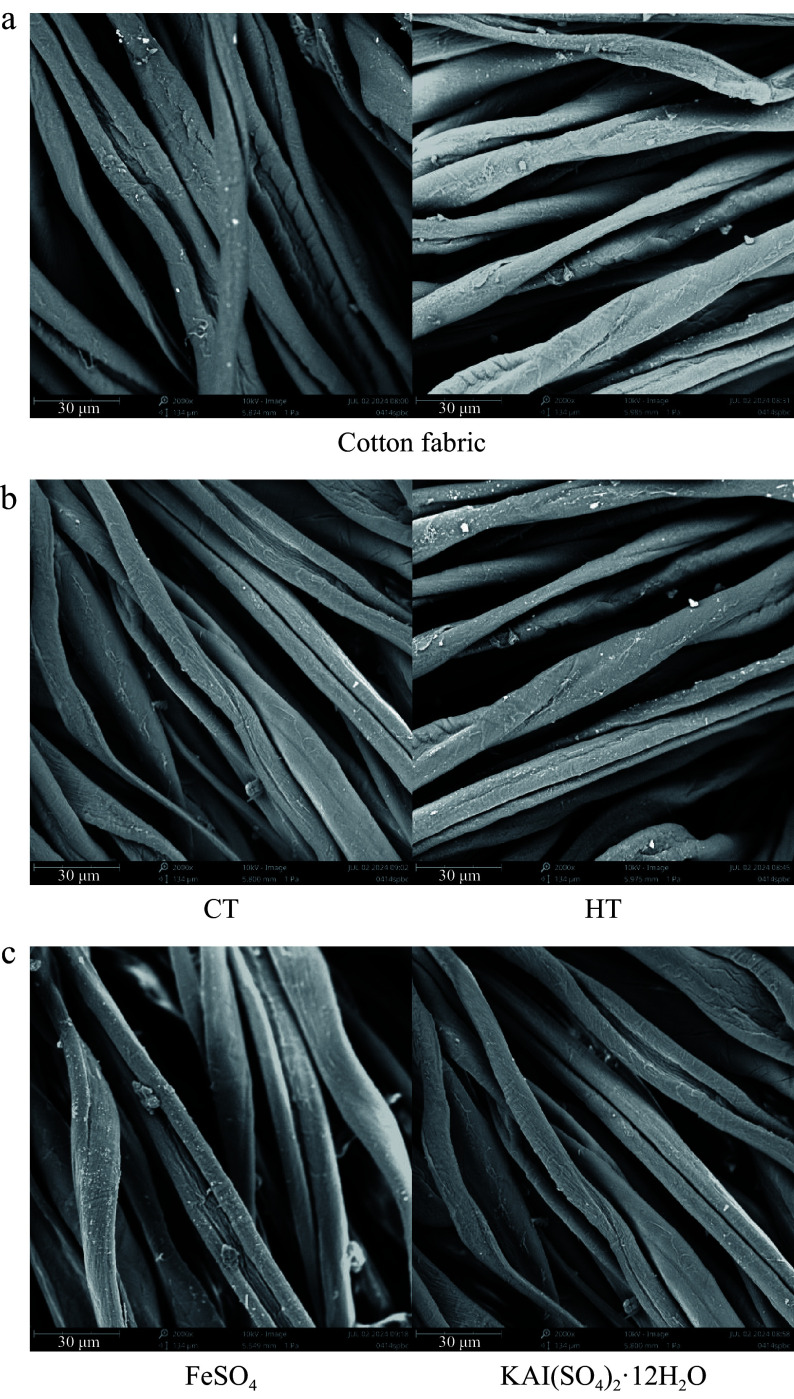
Surface morphology of cotton fabrics. (a) Surface morphology of untreated cotton fabric. (b) Surface morphology of cotton fabric directly dyed with different plant dye components from *Camphora officinarum* seeds. CT refers to the dye solution extracted using 60% ethanol as the solvent, with condensed tannins as the main component, while HT refers to the dye solution extracted using water as the solvent, with hydrolysable tannins as the main component. (c) Surface morphology of cotton fabric after post-mordanting with different mordants using plant dyes from *C. officinarum* seeds.
